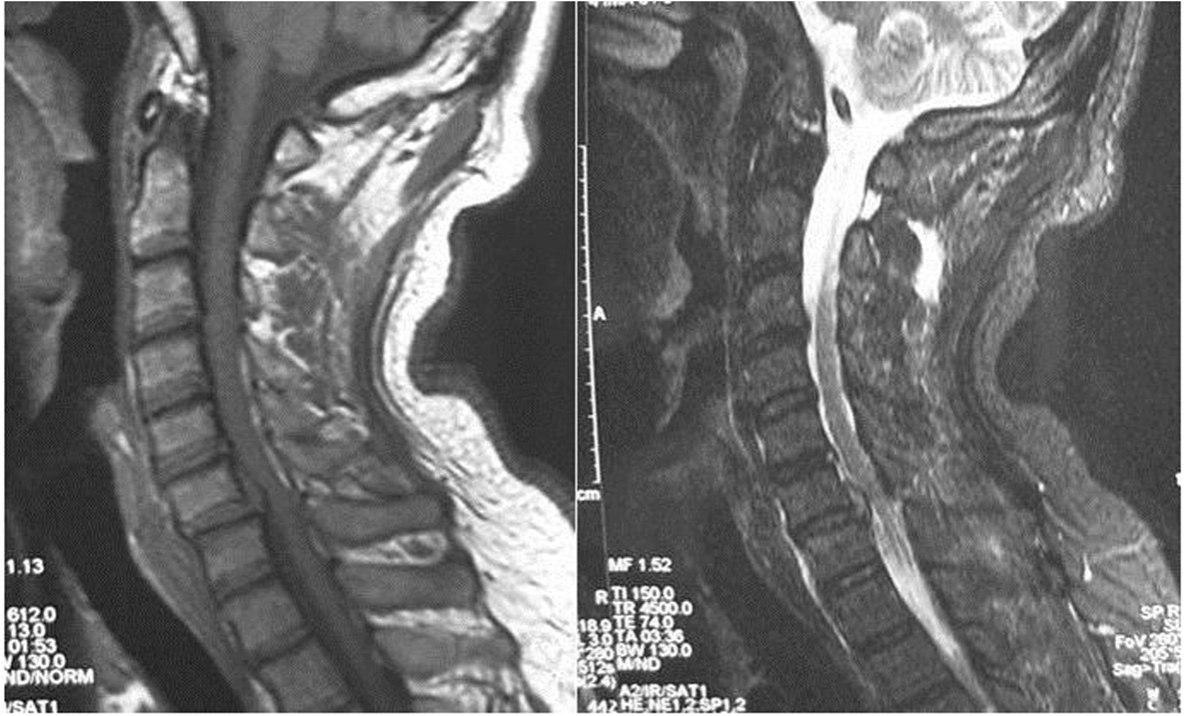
T1-weighted (left) and T2-weighted (right) images demonstrate C6-C7 with Modic-1 changes on MRI.
